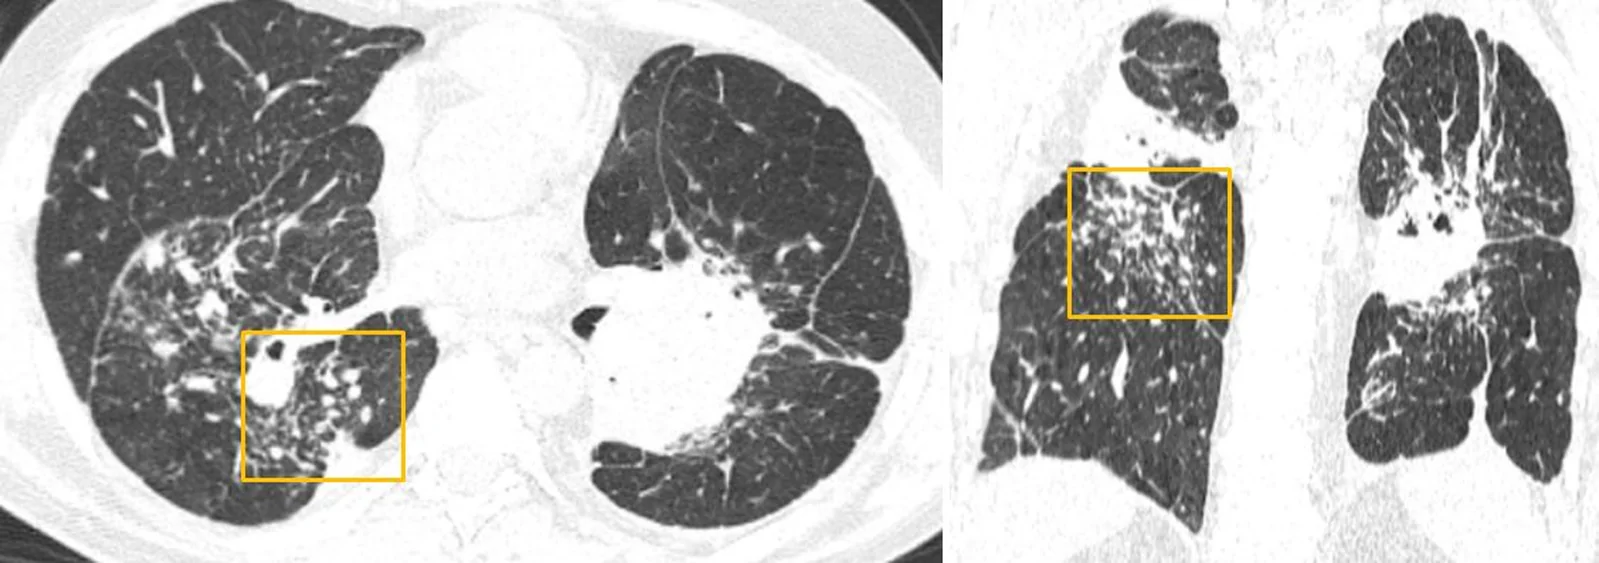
Axial and coronal HRCT images (lung window) demonstrating multiple polymorphic nodules (yellow box) distributed along the bronchovascular bundles and interlobar pleura, without evidence of coalescence. HRCT: high-resolution computed tomography.
